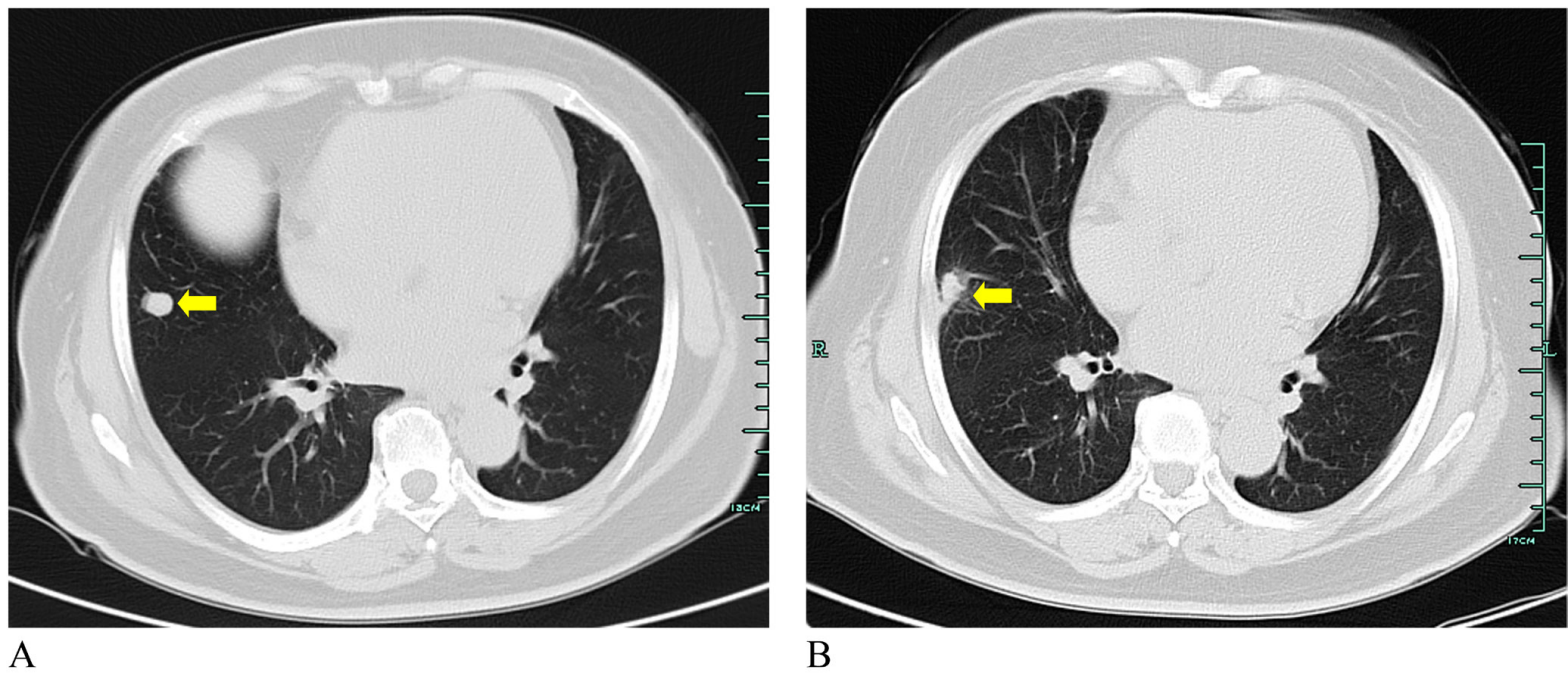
CT images from a 63-year-old woman with lung metastasis from sigmoid colon cancer Coronal unenhanced CT images indicated the decreased tumor after PLA for lung metastasis (A. Before ablation; B. After ablation) (yellow arrows).
